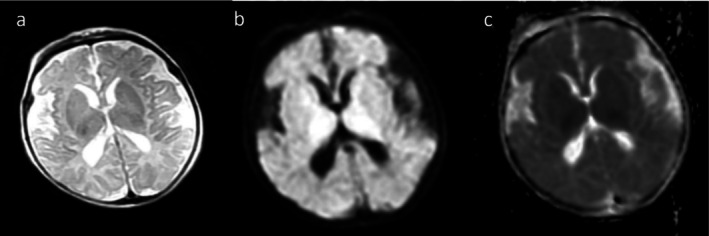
Brain MRI; (a) Axial diffusion‐weighted imaging demonstrates a small hyperintense diffusion‐restricted focus in the left lateral thalamic region. (b) The corresponding Apparent Diffusion Coefficient (ADC) map shows a hypointense signal consistent with true diffusion restriction. (c) Axial FLAIR sequence reveals bilateral frontotemporal atrophy, corpus callosum hypoplasia, and mild enlargement of the lateral ventricles.
